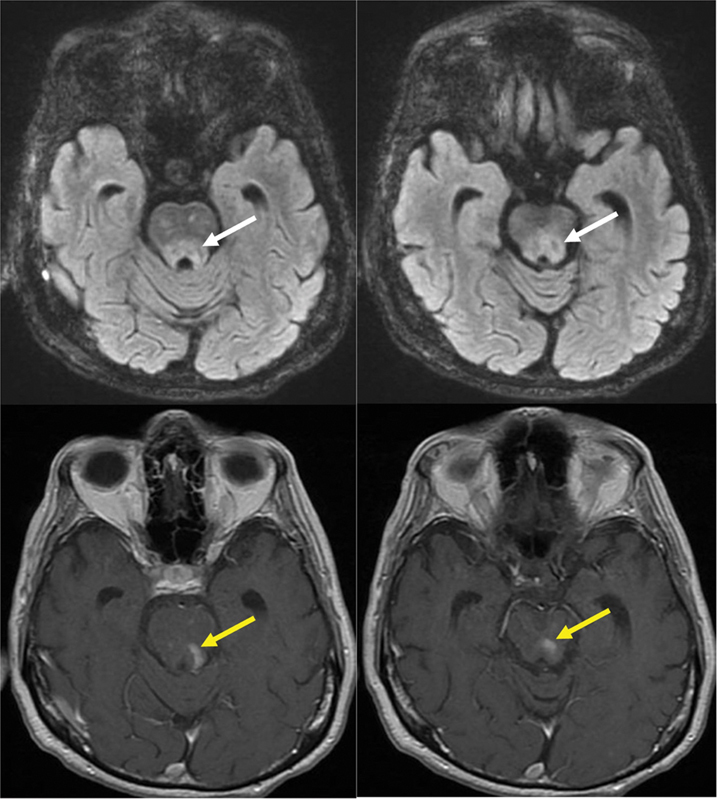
Brain magnetic resonance imaging showed a T2/fluid-attenuated inversion recovery brainstem lesion at the dorsal pontomesencephalic junction (white arrows) with contrast enhancement (yellow arrows).
